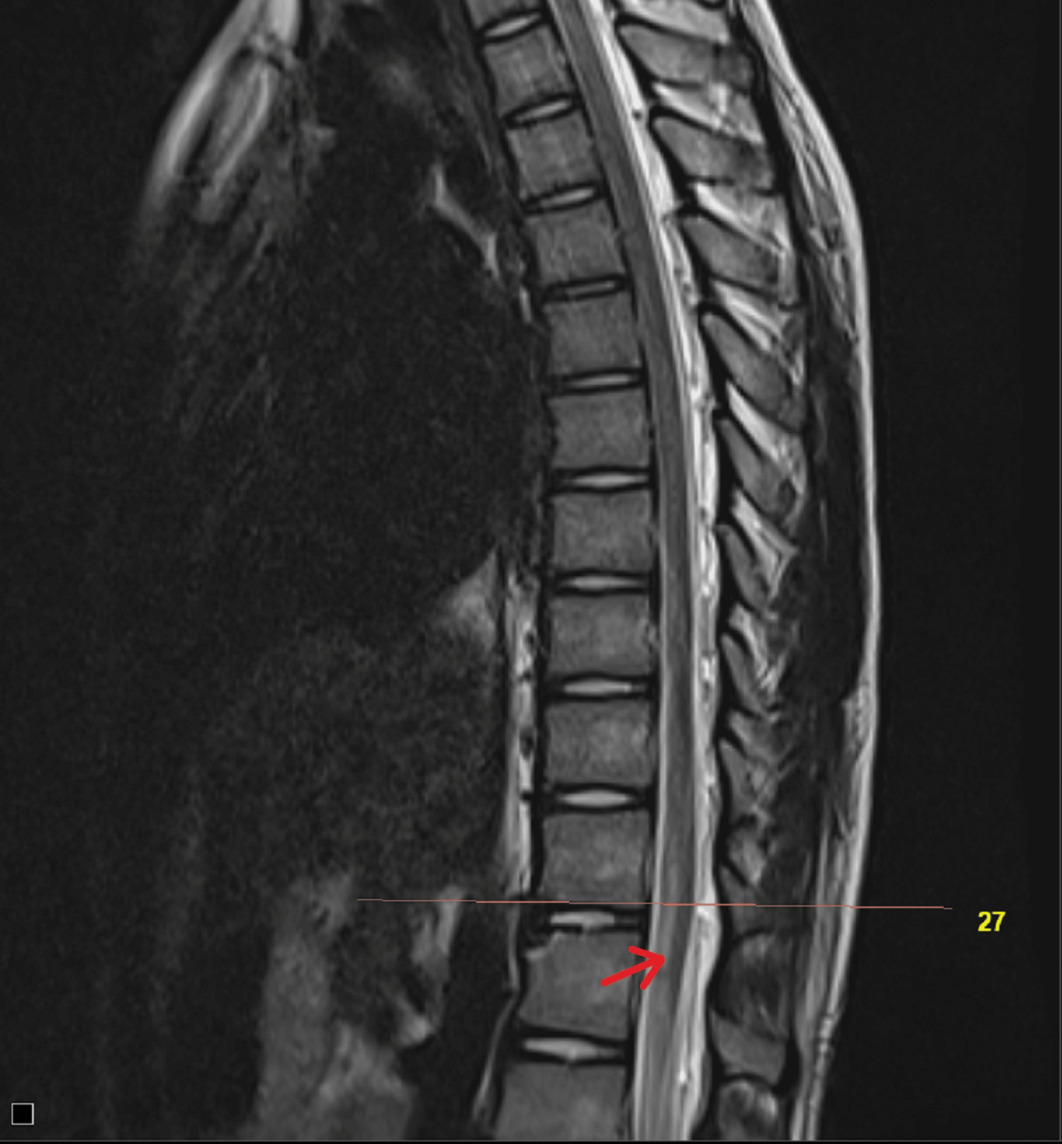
MRI T2 sagittal image of dorsal spine showing ASA syndrome MRI - T2 sagittal image of dorsal spine showing longitudinal ventral cord hyperintensity at D11 to L1 level (red arrow). MRI: Magnetic resonance imaging; ASA: Anterior spinal artery
